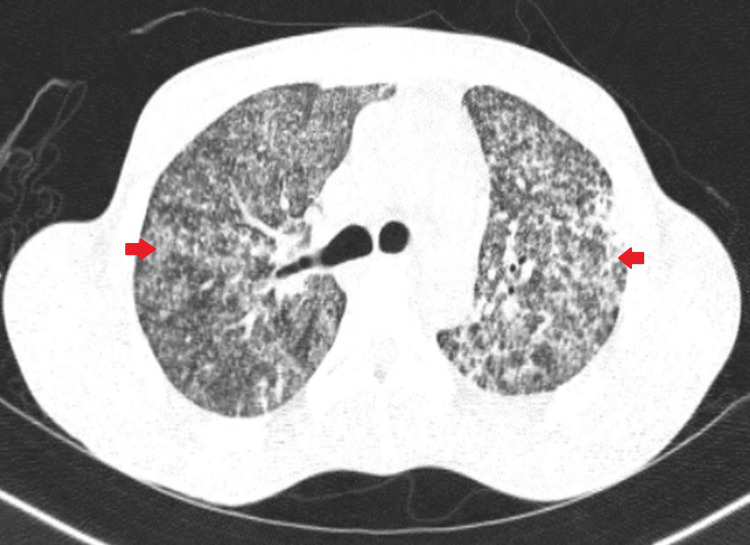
Contrast-enhanced computerized tomography (CT) scan of the chest showing bilateral multiple centrilobular nodules with tree-in-bud appearance (red arrows).
